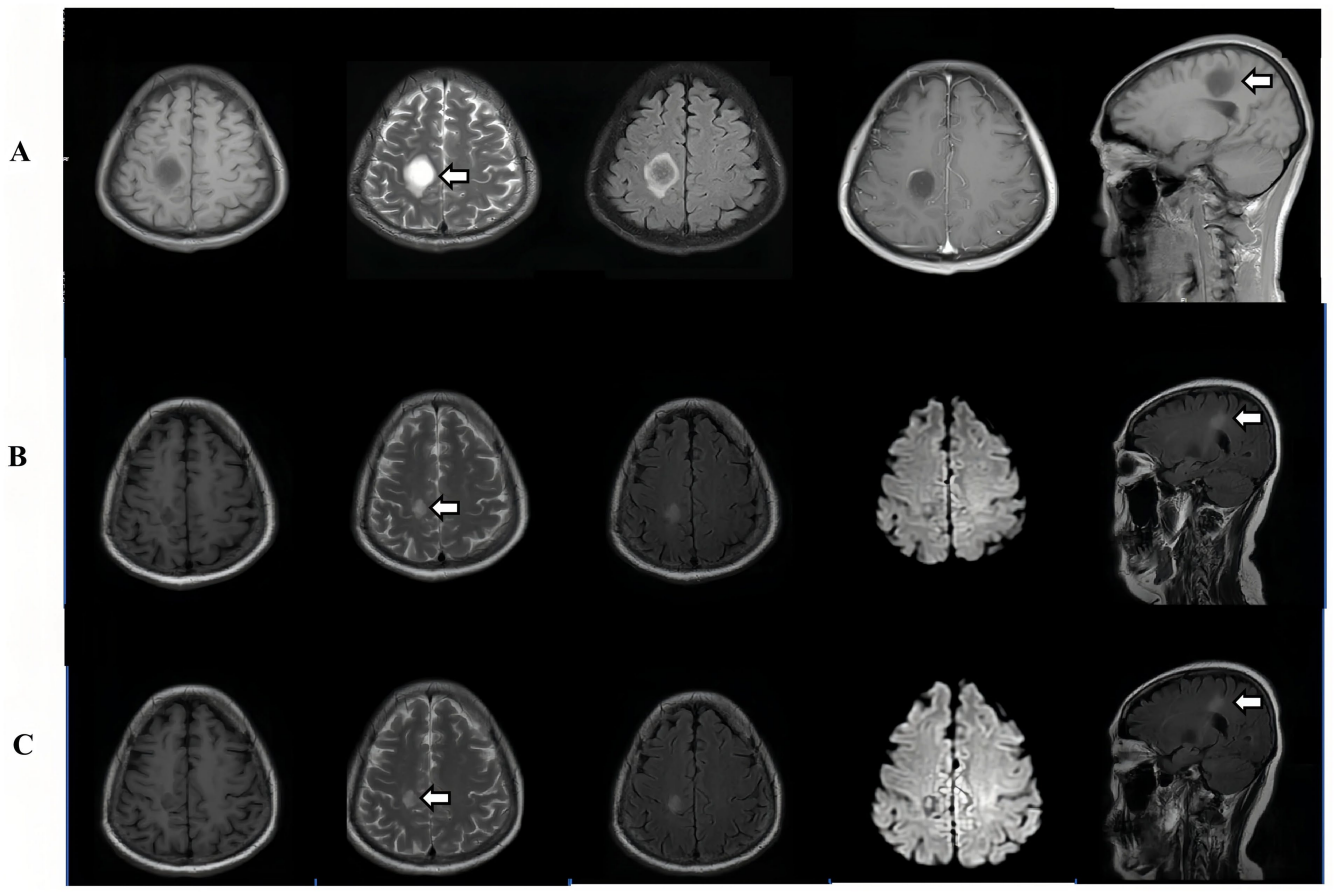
Temporal evolution of brain lesions on MRI. (A) (March 2024) Multisequence brain MRI (T1-weighted, T2-weighted, FLAIR, and gadolinium-enhanced sequences) showed a prominent lesion in the right cerebral hemisphere, with characteristic signal abnormalities and ring-like contrast enhancement (white arrow indicates the core lesion at initial onset). (B) (October 2024) Follow-up MRI demonstrated partial resolution of the lesion, with reduced signal heterogeneity and diminished contrast enhancement (white arrow indicates the partially resolved lesion after treatment). (C) (January 2025) The latest imaging revealed further evolution, with the lesion transforming into a more homogeneous plaque and marked reduction of contrast enhancement, indicating disease progression and modulation over time (white arrow indicates the evolved lesion at relapse).
